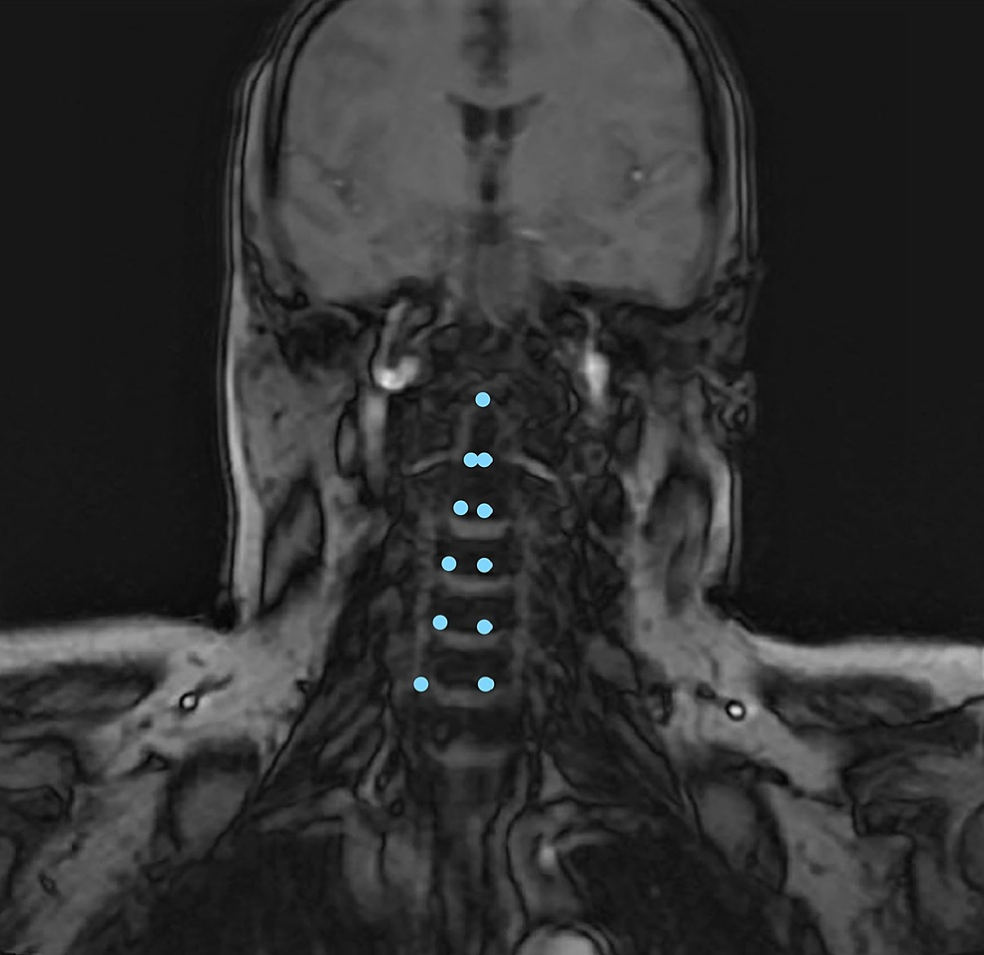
The displacement of the trachea to the right lateral (angular pointed lines), the coronal section, diagnostic imaging of the neck MRI. MRI: magnetic resonance imaging.
